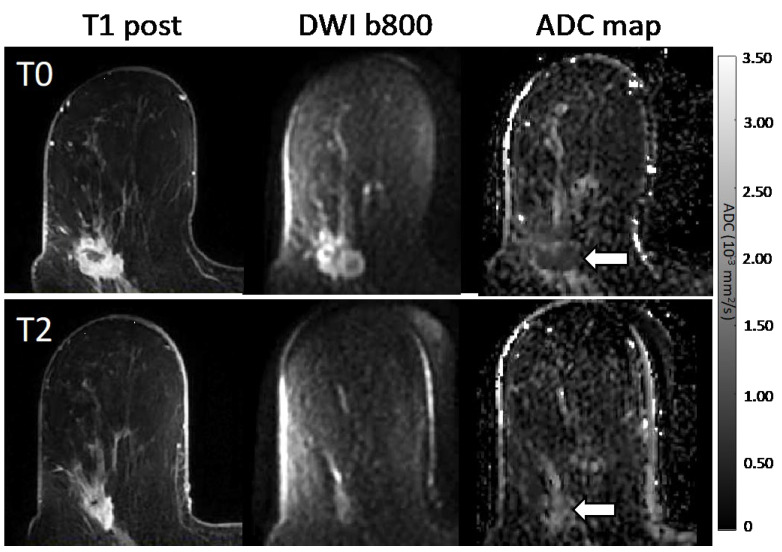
Serial MR images in a 58-year-old patient with a 3.7 cm HR+/HER2− high-grade invasive ductal carcinoma with pCR outcome. Shown are representative matched slices from the dynamic contrast-enhanced (DCE) early post-contrast scan, diffusion-weighted image (DWI; *b* = 800 s/mm^2^), and quantitative apparent diffusion coefficient (ADC) map for MRI examinations at the pre-treatment (T0, top) and mid-treatment (T2, bottom) timepoints. Between T0 and T2, the subject showed a decrease in tumor volume on DCE from 7.0 cc to 1.2 cc between timepoints, and the mean tumor ADC increased from 1.00 to 1.81 × 10^−3^ mm^2^/s.
